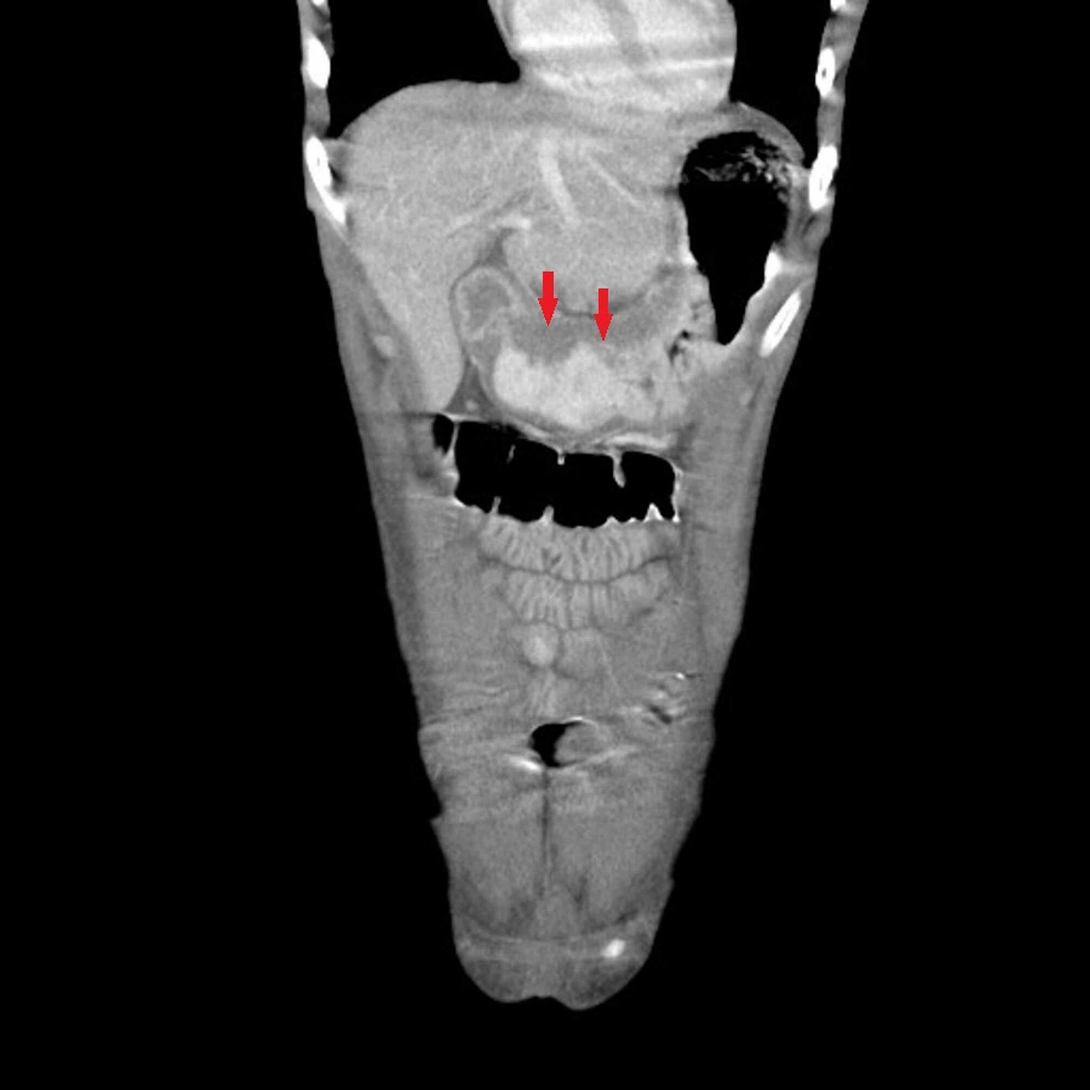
CT scan coronal view: arrows indicate extensive infiltration of the stomach wall with the malignant process
